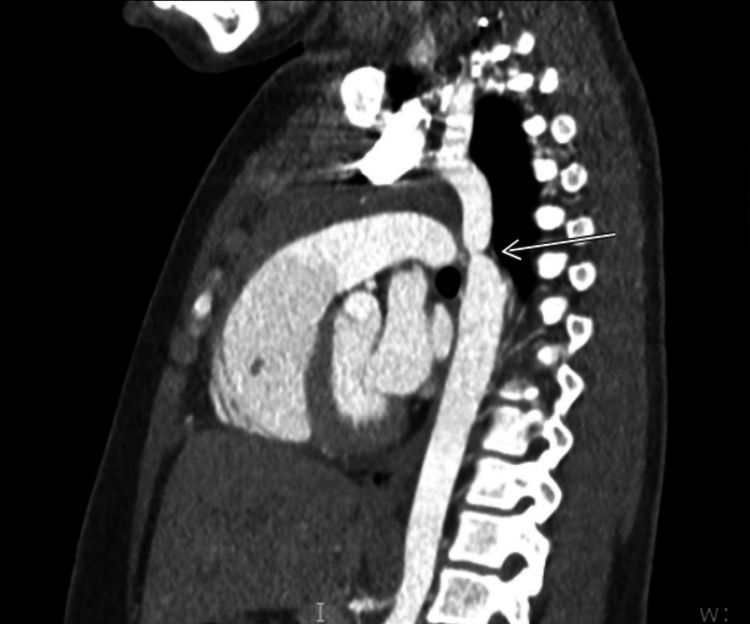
CT angiography The thoracic aorta shows narrowing of the proximal thoracic descending aorta measuring 10 mm in transverse diameter.
